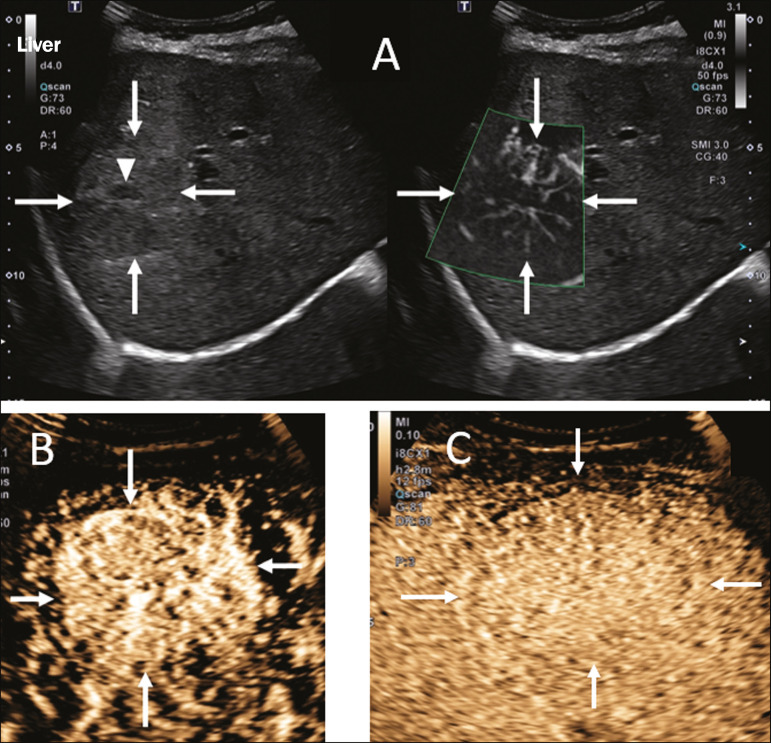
A 14-year-old patient with a liver nodule identified as an incidental finding on ultrasound. A: Simultaneous B-mode and Doppler intensity ultrasound images (left and right, respectively), showing a nodule (arrows on the left) that is slightly hypoechoic in relation to the liver parenchyma, with a hypoechoic center (central scar; arrowhead on the left). On the color Doppler image, a central artery appears as a star-shaped image (arrows on the right). B: After administration of the contrast medium, ultrasound showed a central artery in a star shape (arrows). C: Subsequent centrifugal enhancement, with complete contrast filling and persistence in the delayed phases (arrows).
